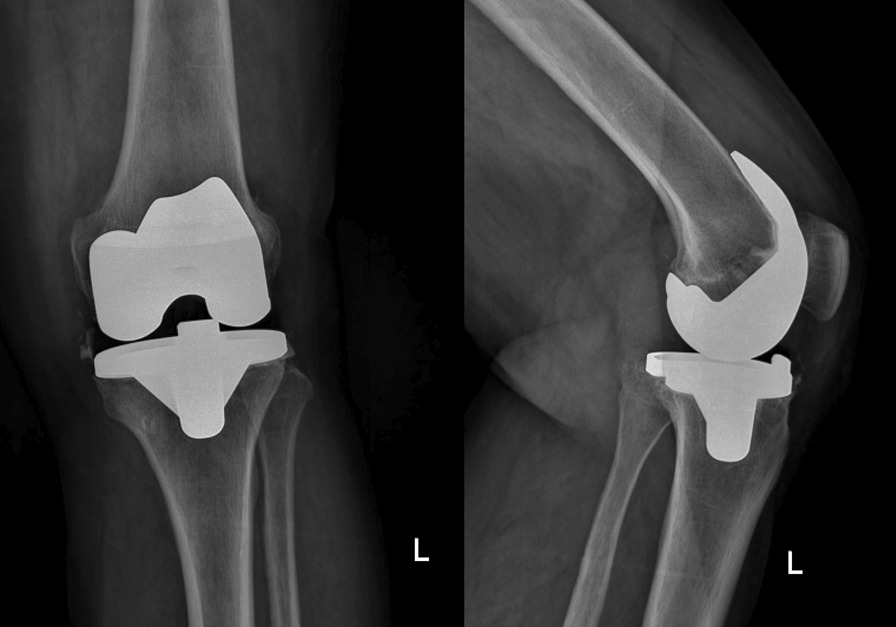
Radiographic image of the implement in the left knee
